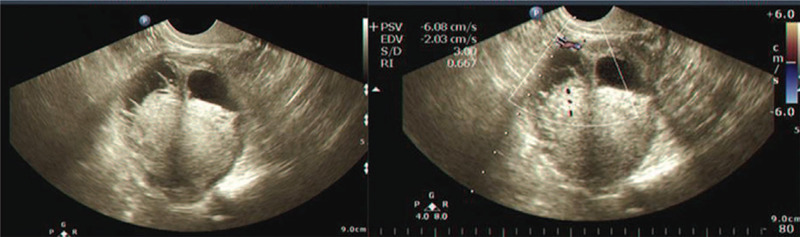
Ultrasound showed that in the left ovarian there was a mass consist of cystic and solid tissue with irregular shape, clear boundaries, and abundant blood flow signal inside.
